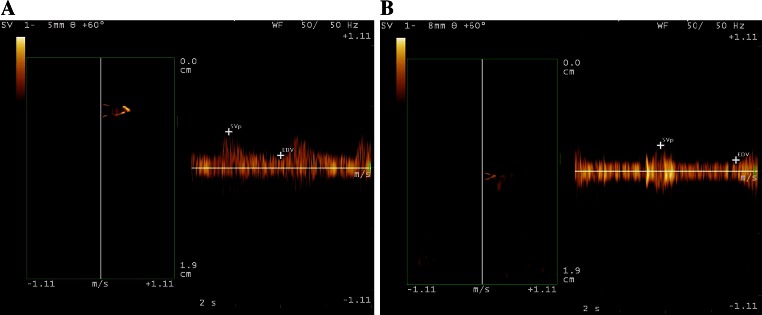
a Shows blood flow profile in the acral finger arterioles obtained by multigated spectral Doppler using QDP technique in a patient with type 2 DM in Group 1 (duration of DM ≤5 years and b shows that in a patient in Group 2 (duration of DM >5 years), in which both the *D* and *A*
